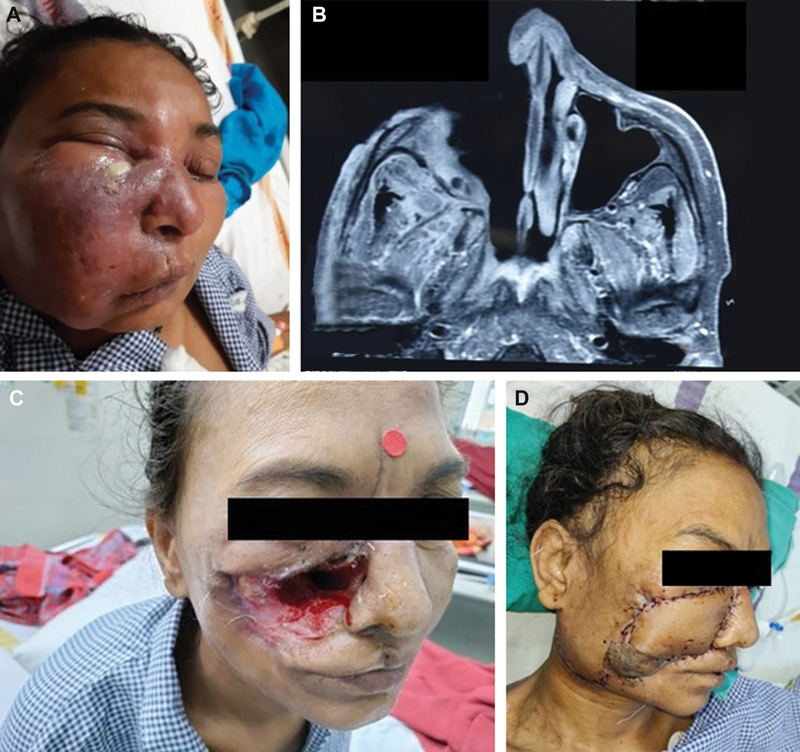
(A) Clinical picture of mucormycosis over right cheek. (B) Magnetic resonance imaging showing the defect in the maxilla. (C) Midface defect after debridement. (D) Defect reconstructed with free radial artery forearm flap.
